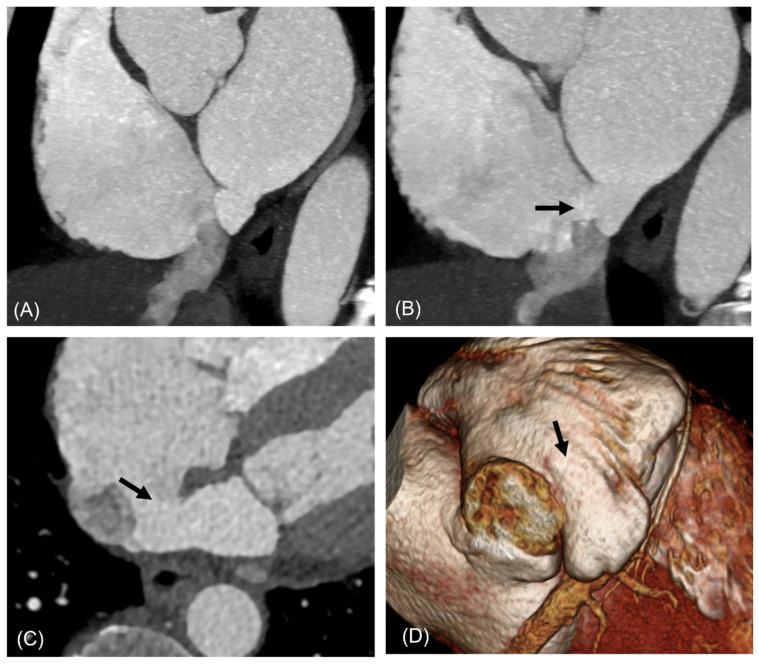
A 76-year-old woman with saccular-type ILAD communicated with RA. Short-axis view of cardiac CT scan shows saccular-type diverticulum originating from the inferior wall of LA (A) communicated with RA (B,C) (arrow). Three-dimensional reconstruction image shows saccular-type diverticulum (D), (arrow). The diverticulum was initially mistaken for an ASD on transthoracic echocardiography, because of its relatively large size and defect. Abbreviations: ASD, atrial septal defect; ILAD, inferior left atrial diverticula; LA, left atrium; RA, right atrium.
